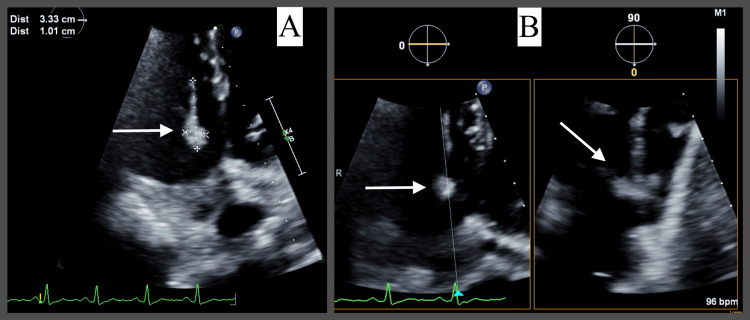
Transthoracic echocardiography (TTE) findings of large, mobile vegetation measuring 3.3 cm x 1 cm (indicated by the white arrows) attached to the tricuspid valve leaflet. A: Apical four-chamber view zoomed on tricuspid valve vegetation. B: Apical four-chamber view with biplane visualization of tricuspid valve vegetation.
